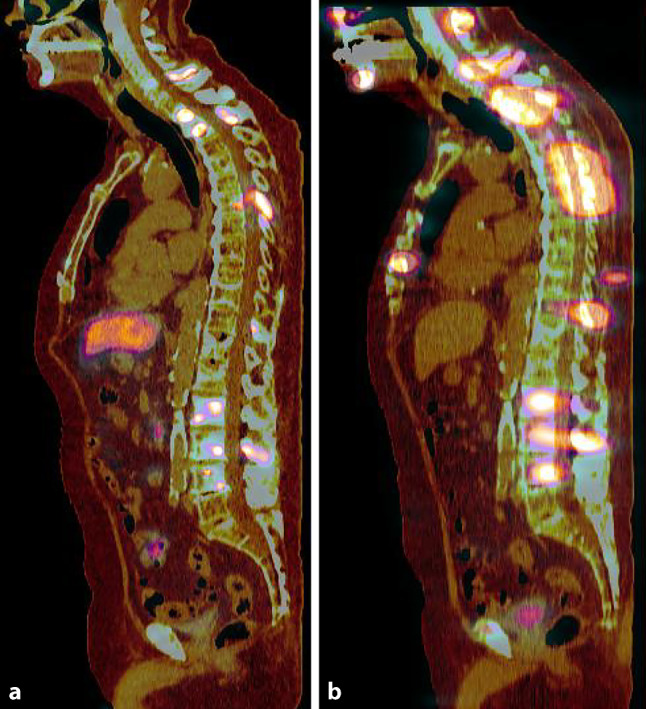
Images of an 83 year-old man with metastatic castration-resistant prostate cancer: ^18^F‑PSMA-PET/CT (a) and ^177^Lu-PSMA-RLT (b) posttherapeutic SPECT/CT (sagittal slice): several foci of PSMA-avid lesions can be seen on both the PET/CT and SPECT/CT images
